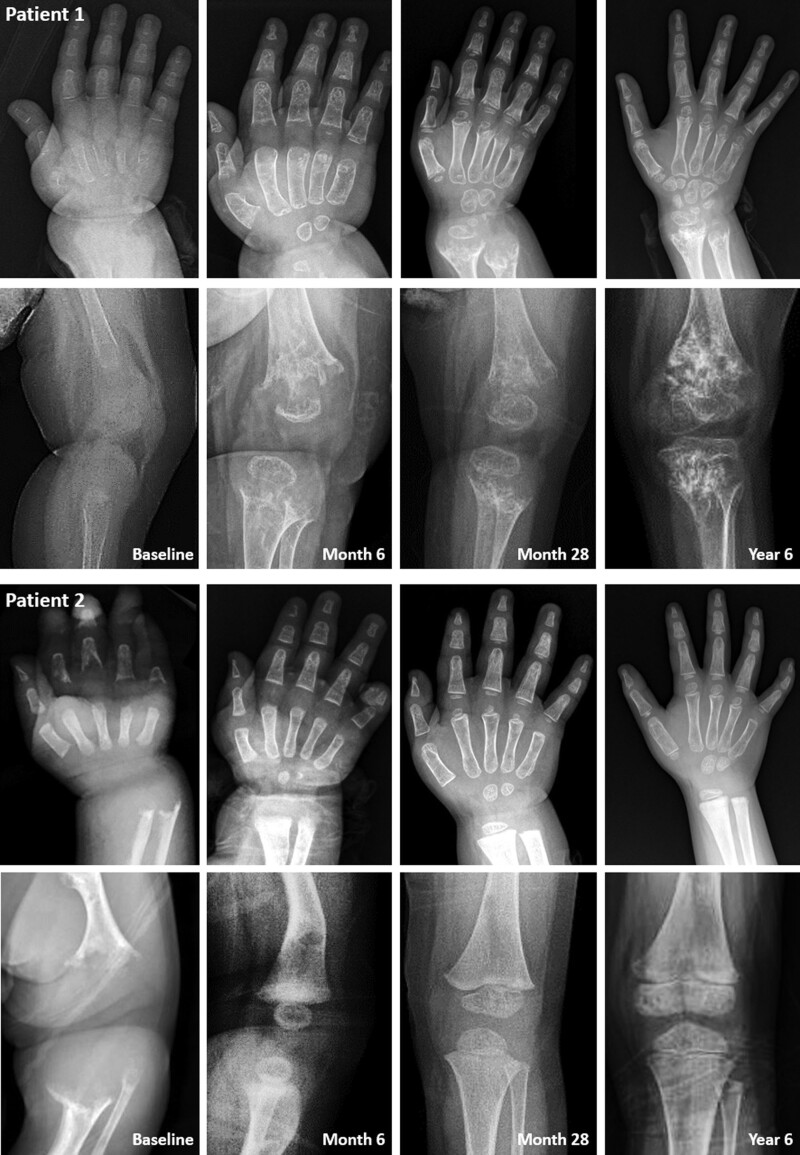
Serial wrist and knee plain radiographs of Patient 1 and 2. The improvement in radiographic findings was more pronounced in Patient 2 compared to Patient 1.
